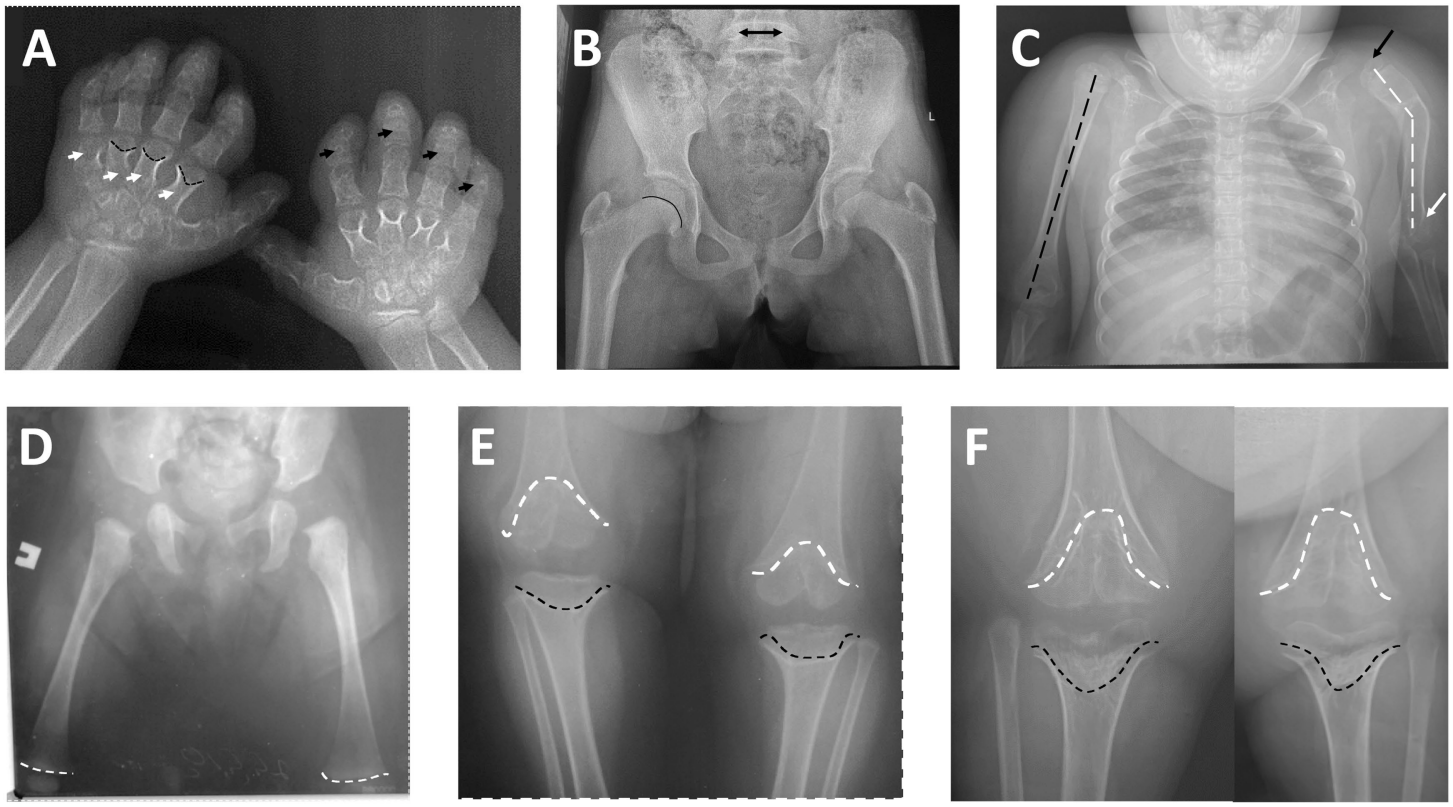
(A) Posteroanterior radiograph of the hands: short metacarpals (white arrows) and phalanges—mostly middle phalanges (black arrows), cone-shaped epiphyses of metacarpal bones (black broken lines). (B) Anteroposterior radiograph of the hips (at 7 years of age)—normal radiographic parameters, intact growth plate (black line) and normal interpedicular distance of L5 (black arrow). (C) Anteroposterior radiograph of the chest and upper limbs: normal axis of the right humerus (black broken line) with intact shoulder and elbow; severe axial deformity of the left humerus (black broken lines), subluxation of the left shoulder (black arrow), and deformity of the left elbow (white arrow). (D) Anteroposterior radiograph of the hips and femoral bones (age 3 months)—notice normal distal femoral growth plates (white broken lines). (E) Anteroposterior radiograph of the knee joints (at 3 years of age): remarkable epi-metaphyseal deformity (cone-shaped) of both distal femur (white broken lines) and proximal tibia (black broken lines). (F) Anteroposterior radiograph of the knee joints (at 7 years of age): progress of the epi-metaphyseal deformity (wedge-shaped) of both distal femur (white broken lines) and proximal tibia (black broken lines).
